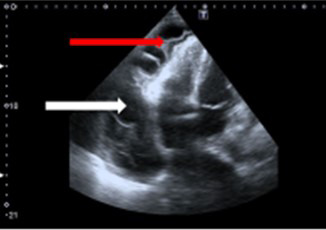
Bedside echocardiography. A modified apical view. White arrow a
